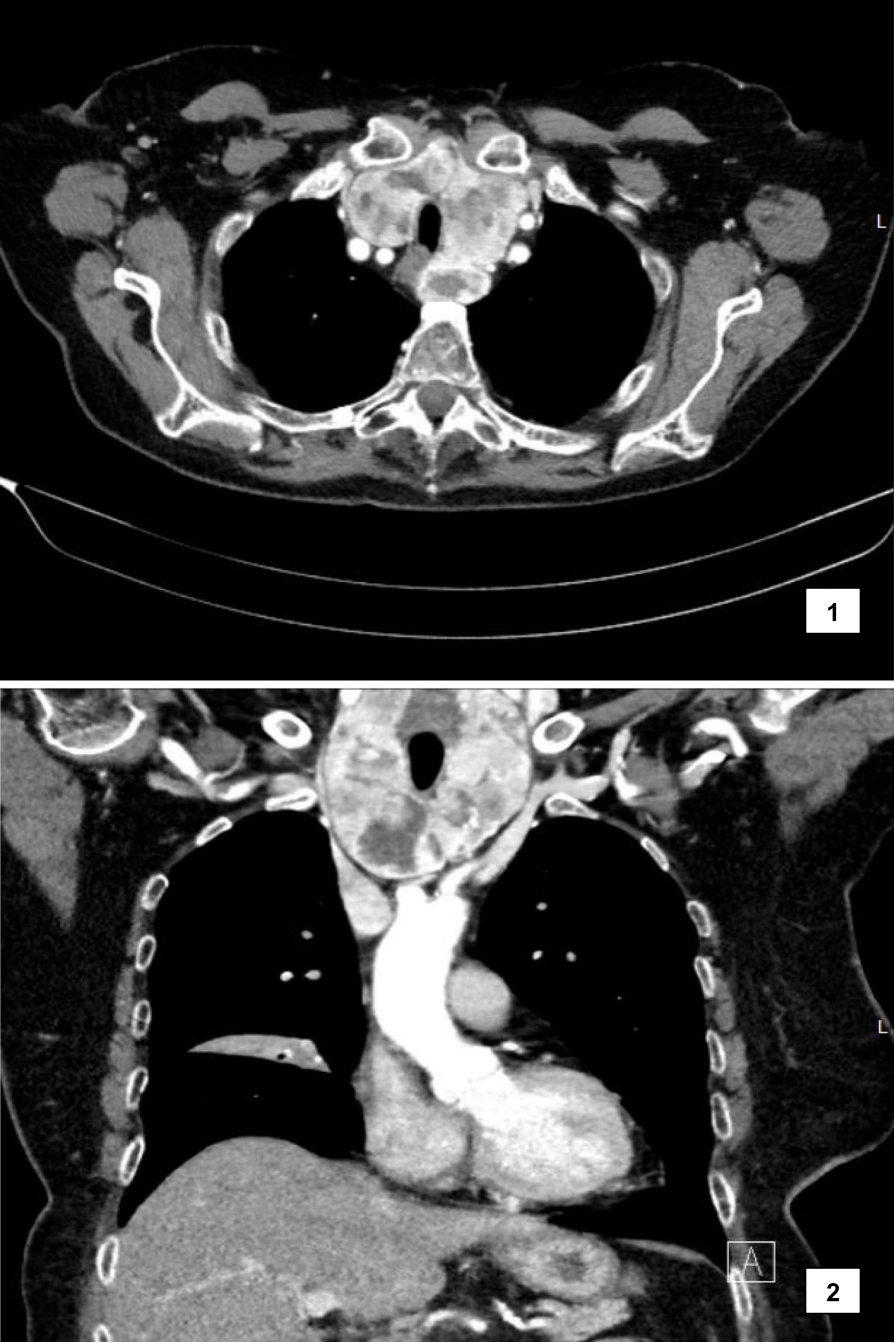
Chest CT revealing multinodular goitre; 1 transverse plane; 2 coronal plane
